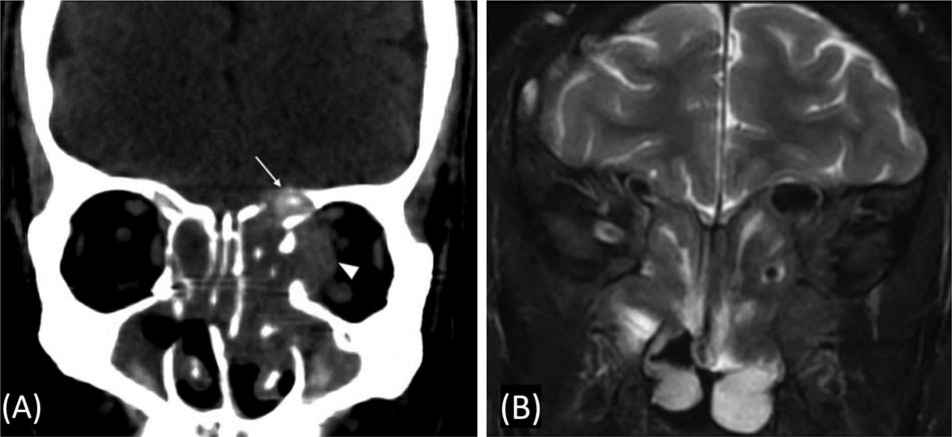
(A) Paranasal sinuses CT scan without contrast showing opacification of the sinuses with hyperdense contents. It results dehiscence of the left lamina papyracea and extension in the medial extraconal space (arrowhead). The ethmoid air cells roof show dehiscence of the roof with intracranial extension (arrow). (B) Coronal T2 MRI through the posterior aspect of left orbit shows the intra-orbital extraconal extension as well as the hypointense left ethmoidal disease with extradural intracranial extension.
